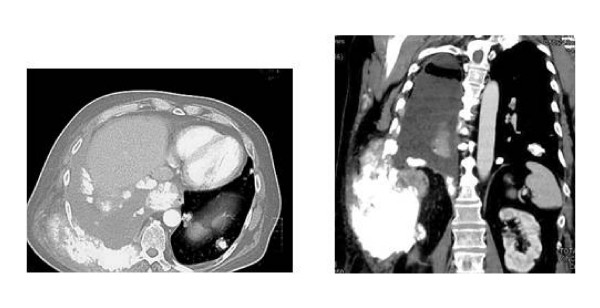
a Thoracic CT scan in the patient with chondrosarcoma shows at right the lesion involving muscles and ribs. Lung metastases were visualized. b Coronal section displays the large tumor.
